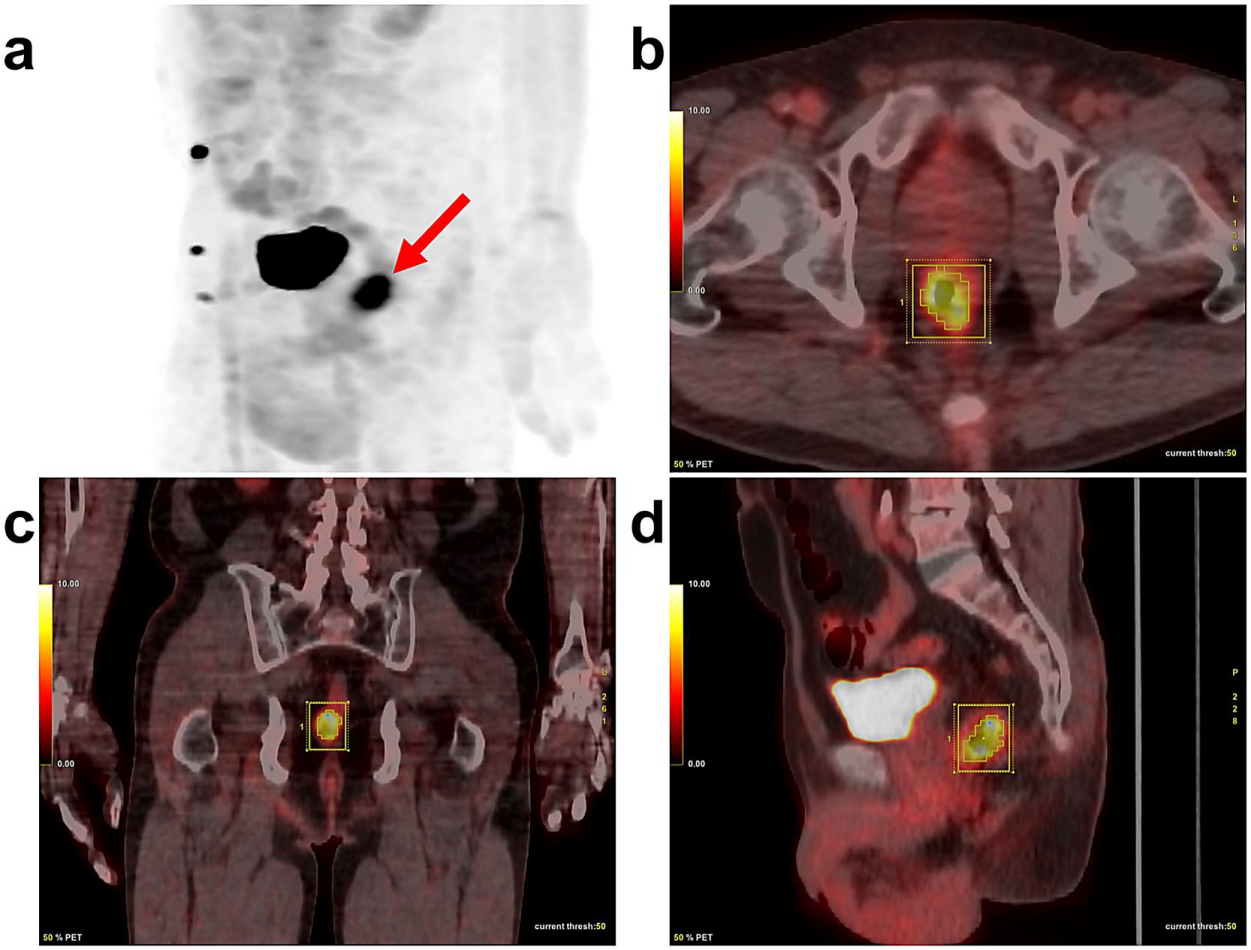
Representative case for the measurement of the maximum standardized uptake value (SUVmax), metabolic tumour volume (MTV), and total lesion glycolysis (TLG) in a 64-year-old male patient with rectal cancer. The maximum intensity projection image shows well-demarcated primary rectal cancer lesion (a) (arrow). We drew a volume of interest to sufficiently surround the primary rectal cancer lesion on transaxial (b), coronal (c), and sagittal (d) images. Then the software automatically calculated the SUVmax (10.40), MTV (7.97 cm^3^), and TLG (56.3 g).
